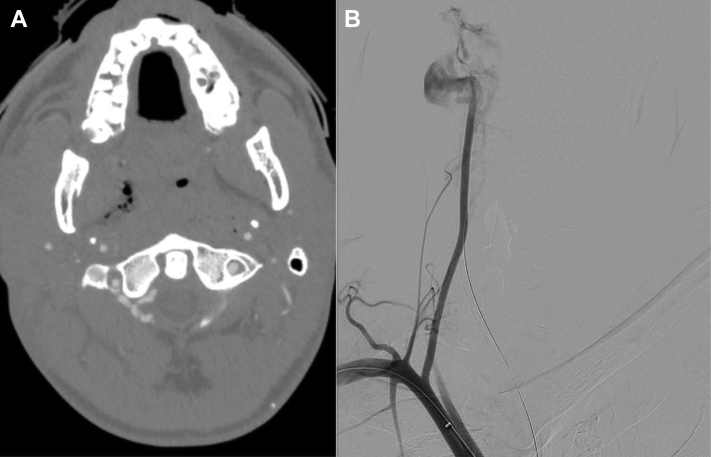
Computed tomography angiogram (A) and arteriogram (B) revealing active extravasation from the right vertebral artery.
